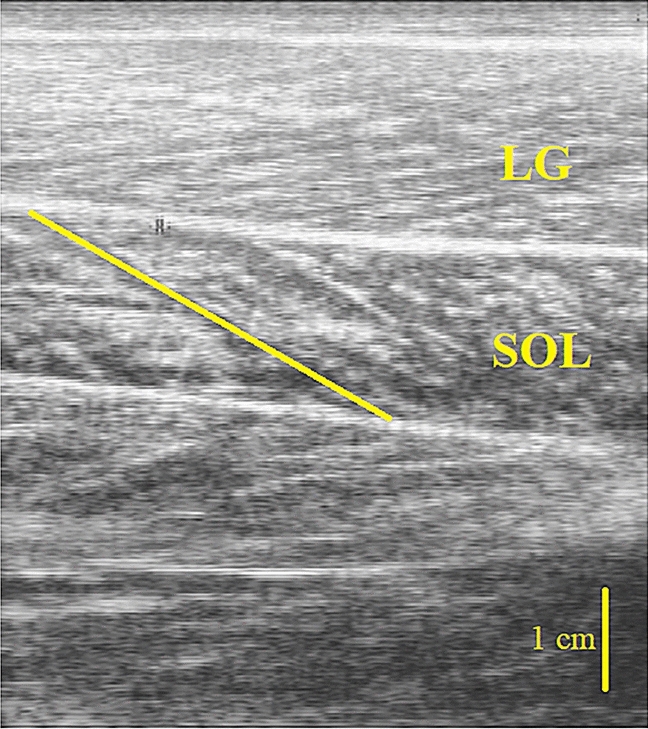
Representative ultrasound image of soleus in sagittal plane for estimate fascicle length. The image was taken at 50% of the muscle length because that region possibly contains the longest fascicles of the muscles. Fascicle length (solid yellow line along fascicles), are drawn in the images.
